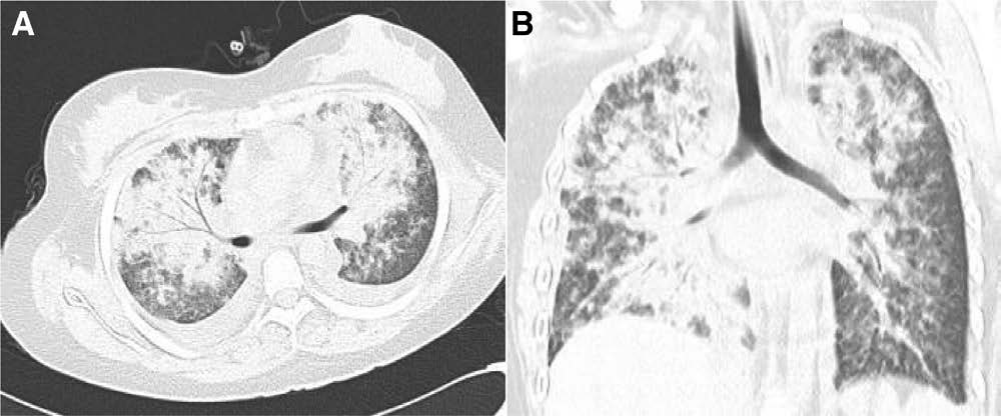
(A and B) Patient P1, female, 16 years old. Chest computed tomography (CT) showed multiple ground-glass opacities in the hilum to the periphery, consolidation, bronchial inflation signs, intralobular interstitial thickening and slight pleural effusion. The chest CT image was not reviewed because the patient died of ARDS. ARDS = acute respiratory distress syndrome.
